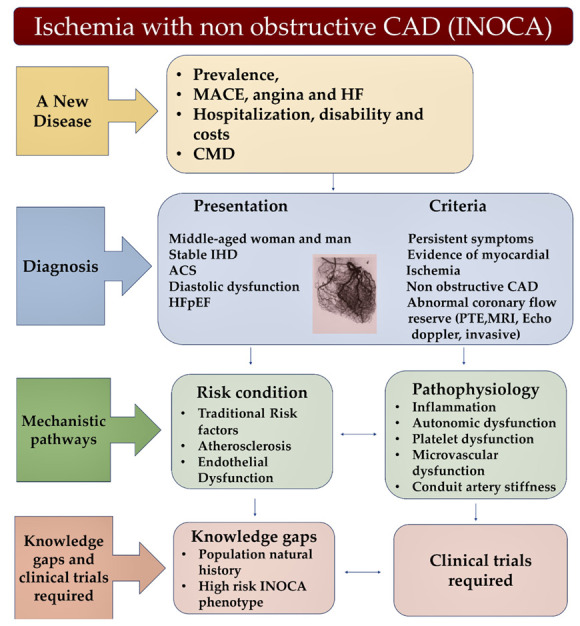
Ischaemia with nonobstructive CAD also called INOCA is a new disease (yellow box) characterized by a specific diagnosis accompanied by typical presentation and criteria (blue box). Risk condition and pathophysiology (green box), as well as an improvement of knowledge (brown box) are of primary importance for the management of INOCA. Abbreviations; IHD; ischaemic heart disease; MRI, Magnetic Resonance Imaging; positron emission tomography; other abbreviations in other figures and table.
